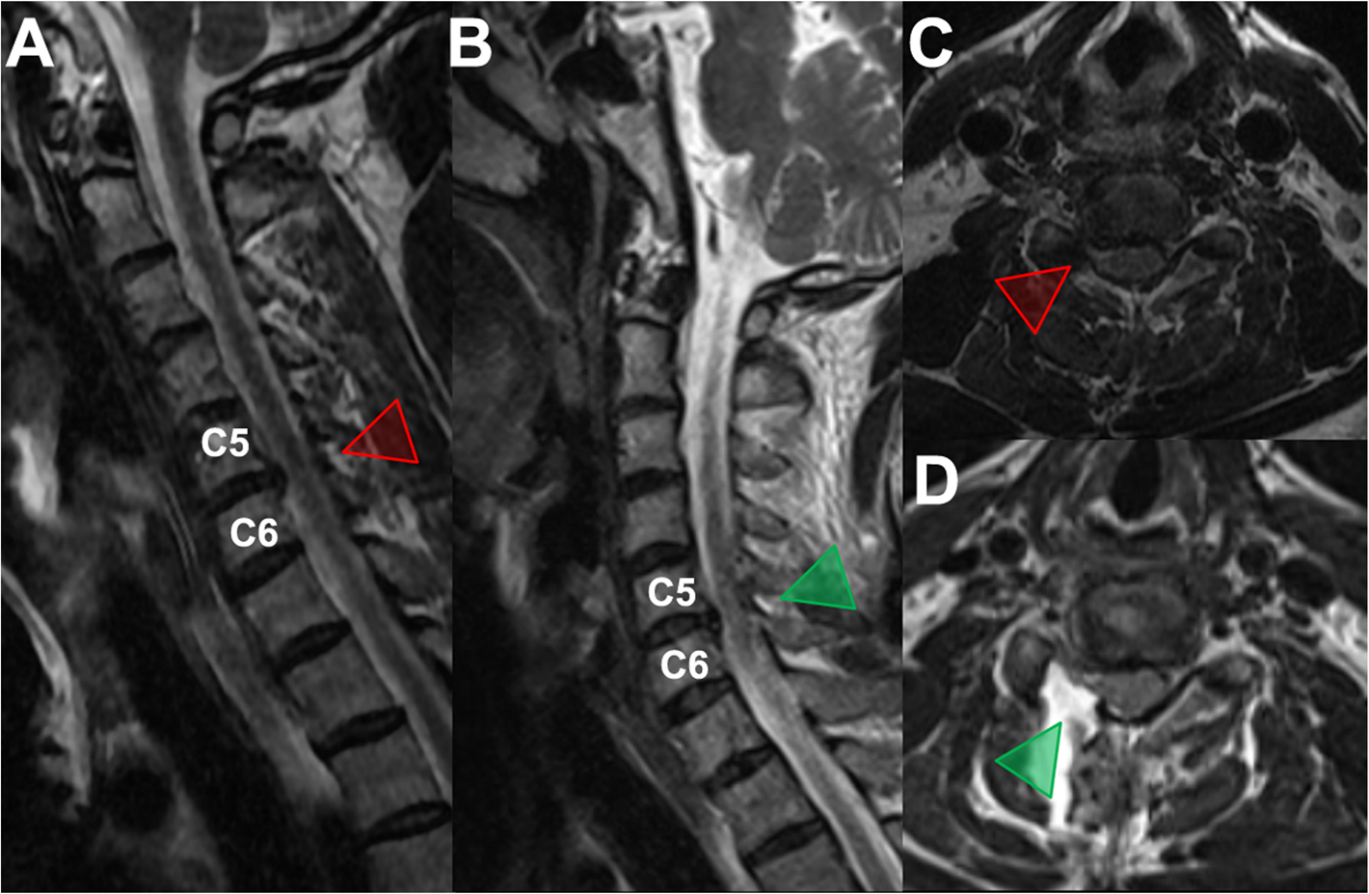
a, c Pre- and b, d postoperative MRI images
